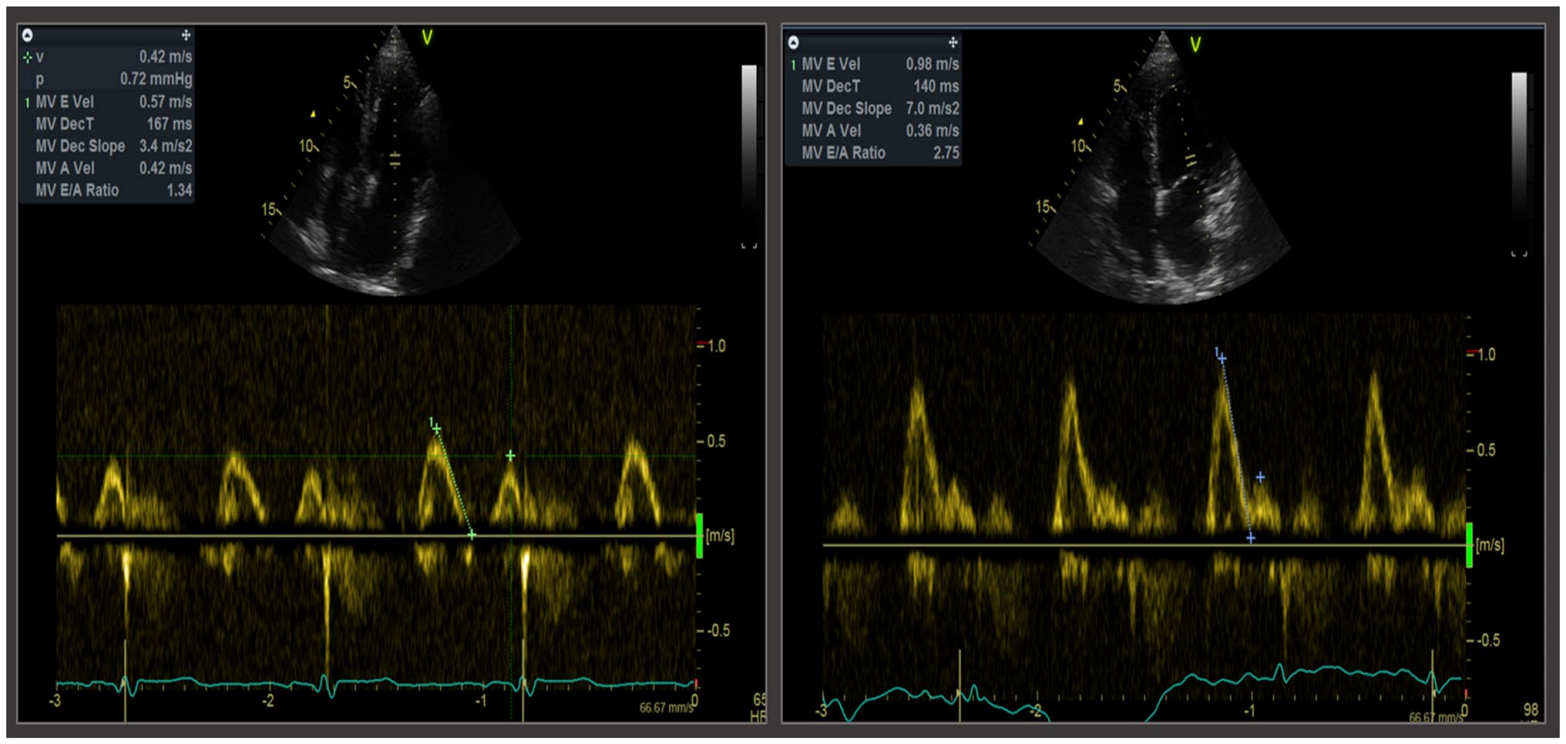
Diastolic dysfunction in presence of cardiac allograft vasculopathy. The left picture shows a normal diastolic function, as indicated by pulsed wave trans-mitral Doppler velocities and deceleration time of E wave, in a heart transplant patient without cardiac allograft vasculopathy. On the other hand, the right picture shows a restrictive diastolic pattern in presence of grade 3 cardiac allograft vasculopathy
